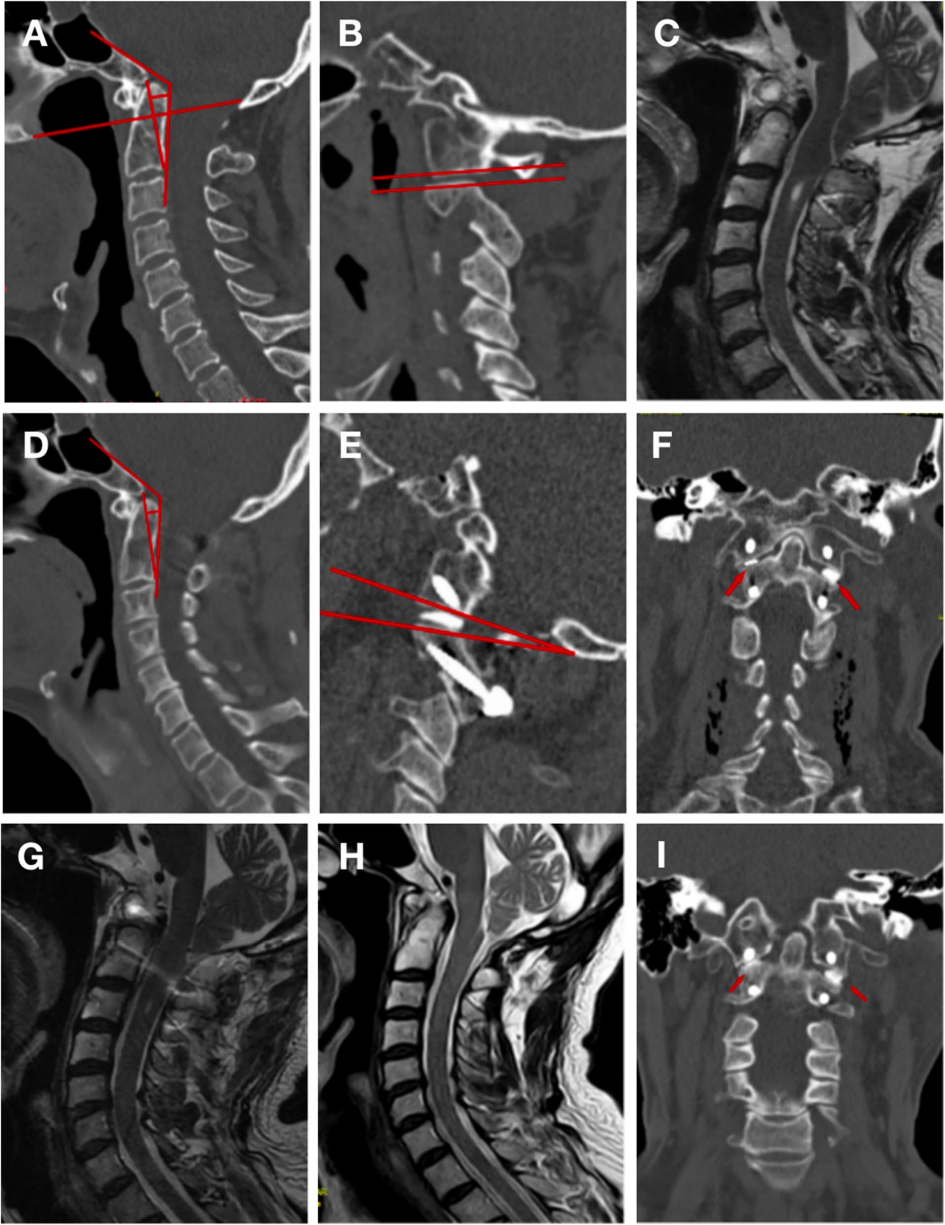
A 51-year-old man presented with gait disturbance and dizziness for six months. The symptoms of the patient significantly improved postoperatively, and the JOA score increased from 13 before the operation to 16. A and B: Sagittal reconstructed computed tomography (CT) scan showing the presence of occipitalization of C1, basilar invagination (BI) without atlantoaxial dislocation (AAD), clivus-canal angle (CCA) = 128.3°, pB-C2 = 5.3 mm, and that the relationship of the two facets of the C1–C2 joint was relatively parallel (red lines). C: Preoperative magnetic resonance imaging (MRI) scan showing associated Chiari malformation and syringomyelia. D: Postoperative sagittal CT showed that the odontoid process decreased slightly and the CCA increased; CCA = 135.6° and pB-C2 = 2.7 mm. The two facets of the C1–C2 joint are not parallel E. F: The facets of C1 and C2 were released, and an autologous bone block was implanted. G: Sagittal MRI showed a slight decrease in the syringomyelia and a slight upward movement of the tonsillar herniation. The 30-month follow-up showed that the syringomyelia disappeared completely and the tonsillar herniation was reduced (H), and bony fusion occurred as confirmed on the CT scan I
